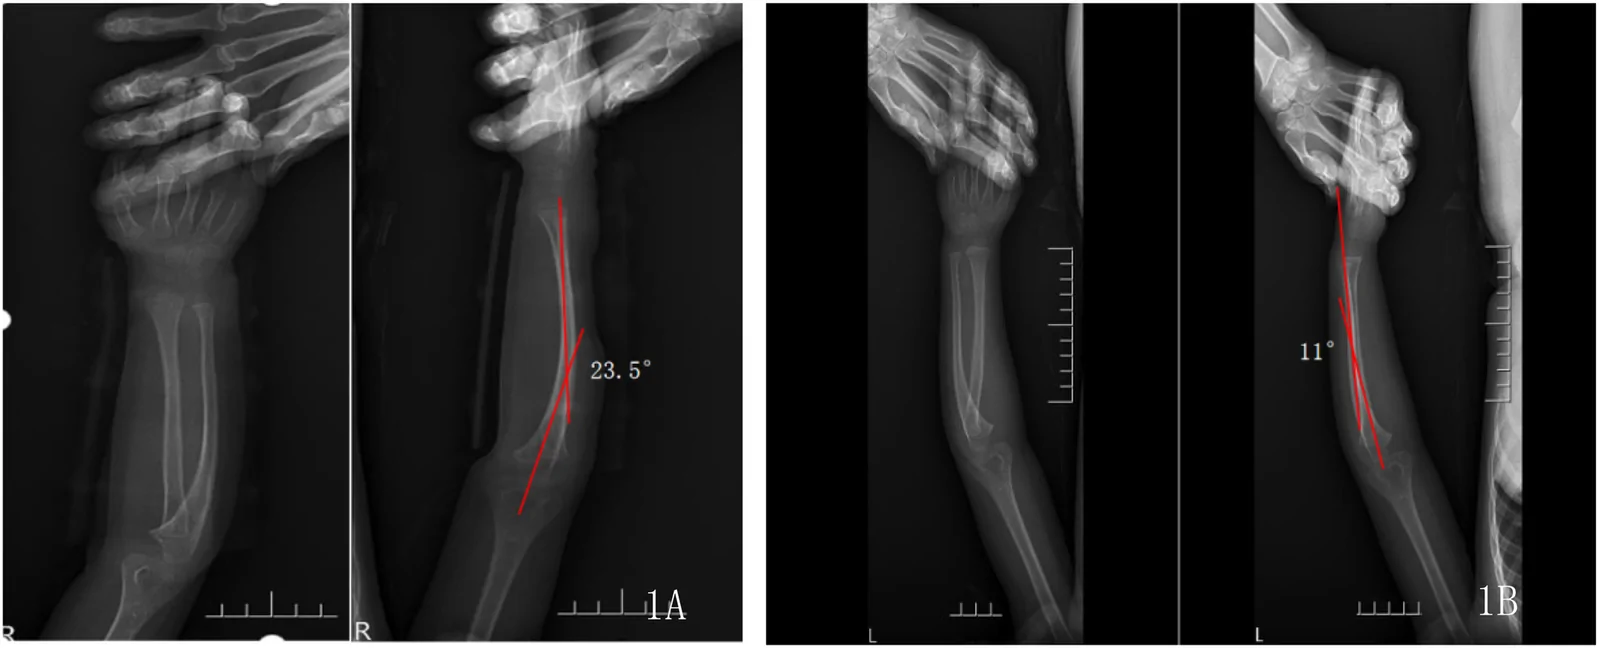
Imaging findings of the affected forearm after initial closed reduction (A); contralateral intact left forearm radiograph (B).
